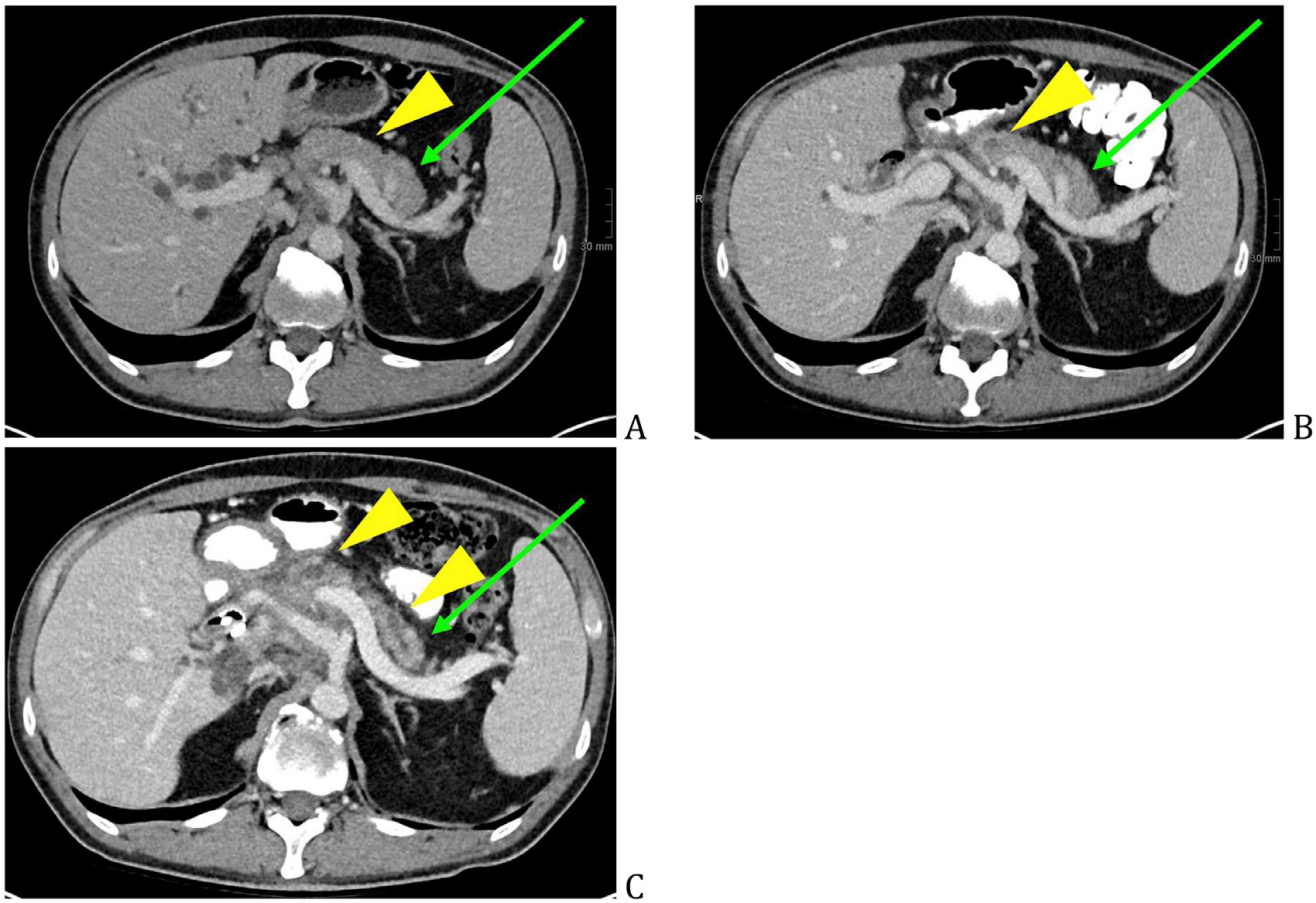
Follow-up CT - span of 4 months, pancreatic tail atrophy (green arrow) and main pancreatic duct upstream dilatation (yellow arrowhead). Initial work-up—no pancreatic atrophy and no duct dilatation (A), follow-up after 1 month—early signs of pancreatic tail atrophy and main pancreatic duct minimal dilatation (B), follow-up after 4 months—evident pancreatic tail atrophy and marked main pancreatic duct dilatation (C).
